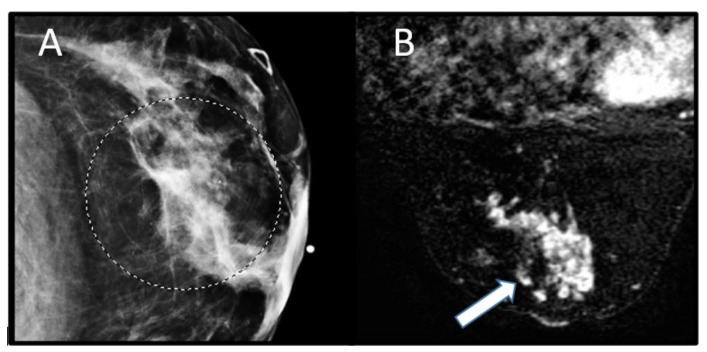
A 37-year-old woman with a clinical diagnosis of Cowden syndrome (negative for *PTEN* mutation) and history of thyroid cancer at age of 24 and Wilms tumor at age 5, presenting with a palpable left breast mass. (A) Mammographic examination demonstrates an irregular mass in the 3 o’clock position centered 1 cm from the nipple with microcalcifications (circle). (B) Postcontrast, fat-saturated magnetic resonance image of the breast shows mass enhancement involving 3 of 4 quadrants due to multiple confluent masses (arrow), which was pathologically found to represent invasive mammary carcinoma with predominantly lobular differentiation and focal ductal component.
